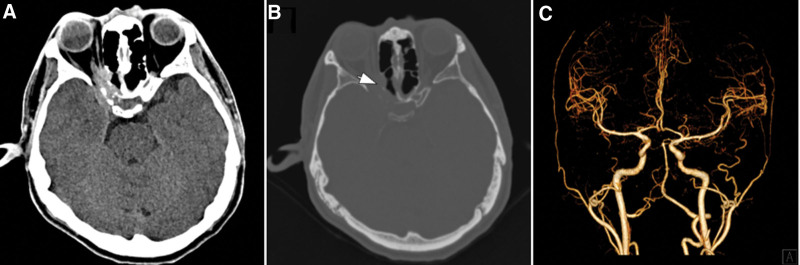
(A) Head CT indicated abnormal signal shadows in the right paranasal sinus considering the possibility of paranasal sinusitis or malignant tumor on first admission. Right posterior ethmoid sinus with bone was destructed in the corresponding areas(arrow indicates). (C) CT angiography indicated no aneurysms or vascular malformations were found.
